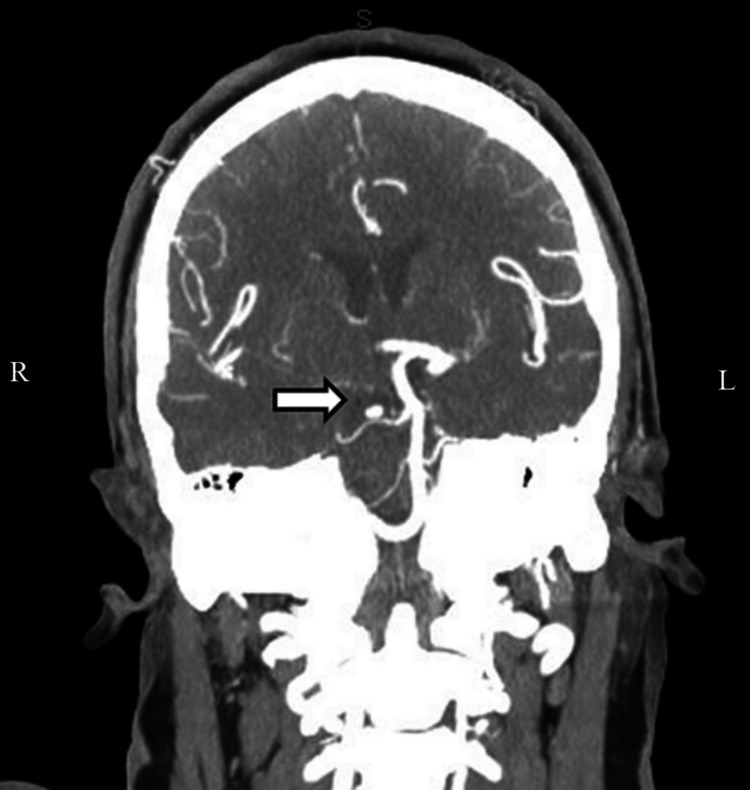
CTA head and neck with contrast - coronal section showing an absence of the right posterior cerebral artery. CTA: computed tomographic angiography.
